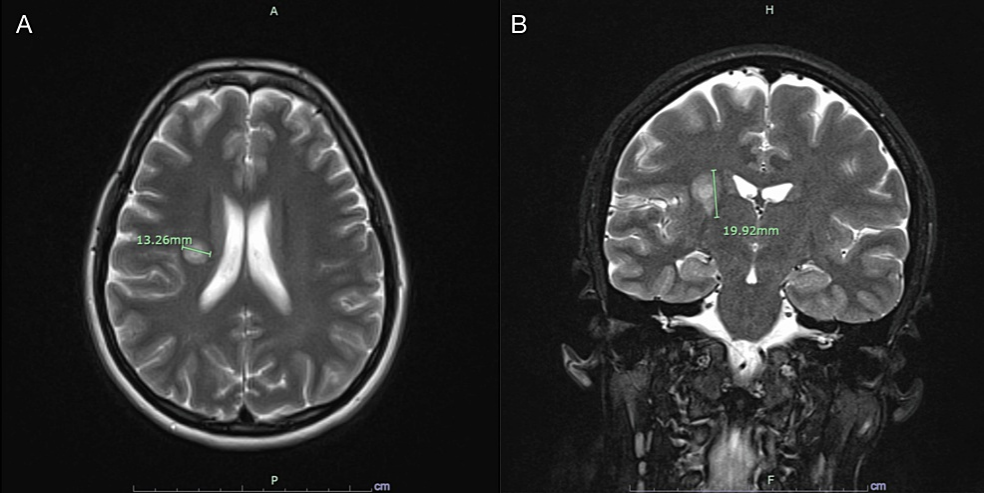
Initial MRI MRI brain demonstrating a solitary BCS-type lesion. Figure 1A displays a T2-weighted axial image showing a well-defined, rounded lesion measuring 13.26 mm in the right corona radiata with alternating layers of high and low signal intensity giving a “bullseye” or “onion bulb” appearance without any significant perilesional edema. Figure 1B displays a T2-weighted coronal image of the same lesion measuring 19.92 mm.
